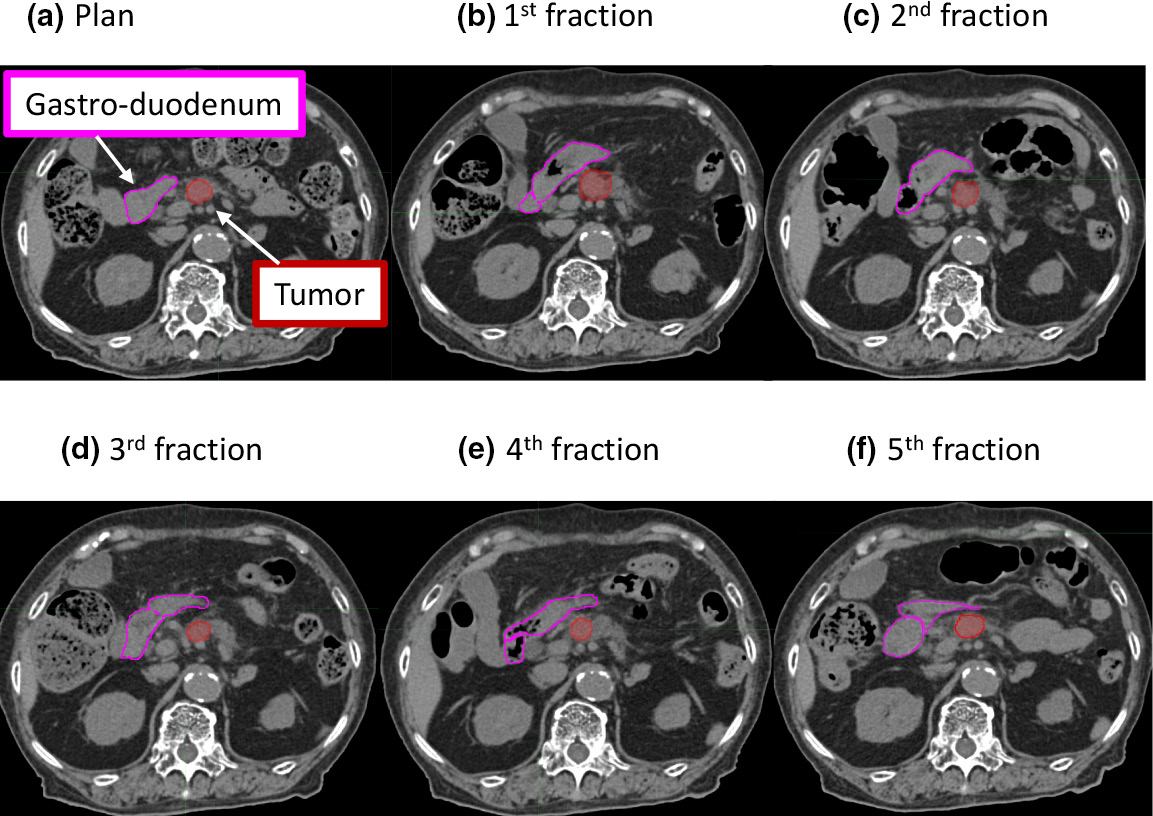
A case showing interfractional motion The gross tumor volume (red) and gastroduodenum (pink) are shown on each CT image. The shape of the gastrointestinal tract is different due to the interfractional motion, leading to daily changes in the shortest distance between the tumor and gastrointestinal tract. CT, computed tomography.
